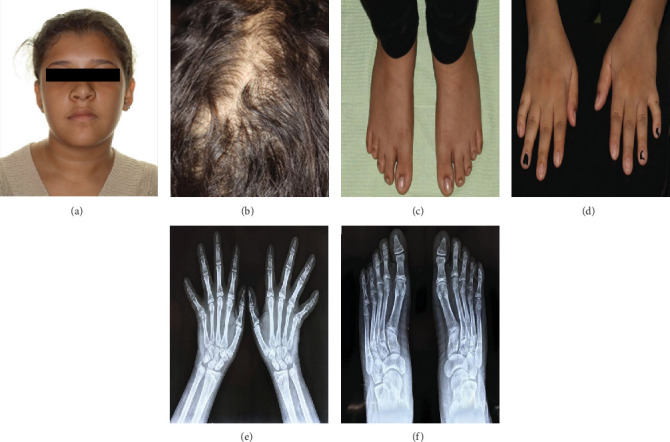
(a) Broad, rounded nasal tip; eyebrows are thicker medially. (b) Thin and sparse hair with areas of the scalp showing. (c) Brachydactyly of the toes. (d) Brachydactyly of the fingers with radial deviation of the distal phalanges of the little finger of both hands. (e) Hand radiography showing brachydactyly, cone-shaped epiphyses, and radial deviation of the little finger. (f) Feet radiography showing brachydactyly of the toes.
